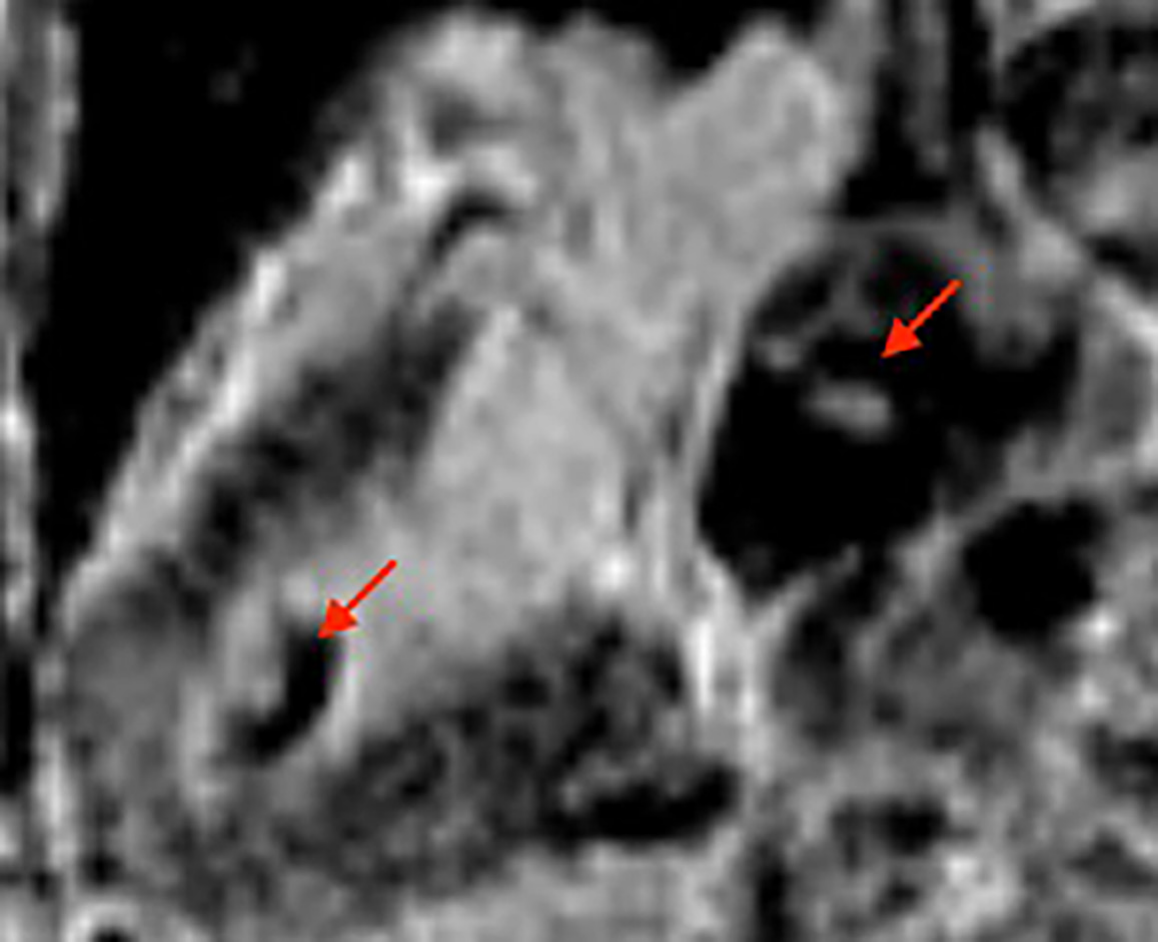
Cardiac MRI post Gd‐contrast IR (inversion recovery) LGE (late gadolinium enhanced) sequences showing peripheral enhancement with necrosis in the center of both masses (arrows). The masses have similar signal characteristics on CMR consistent with their common origin.
